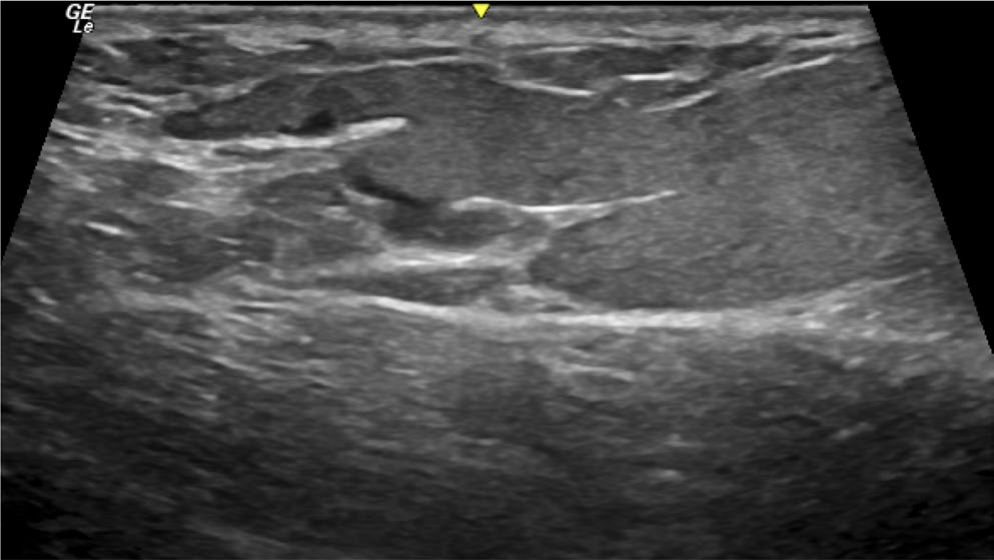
Second polyacrylamide gel. Ultrasound image showing well‐defined hypoechoic collections with internal debris, which may exhibit movement during static maneuvers. Characteristic of PAAG migration and potential complications.
